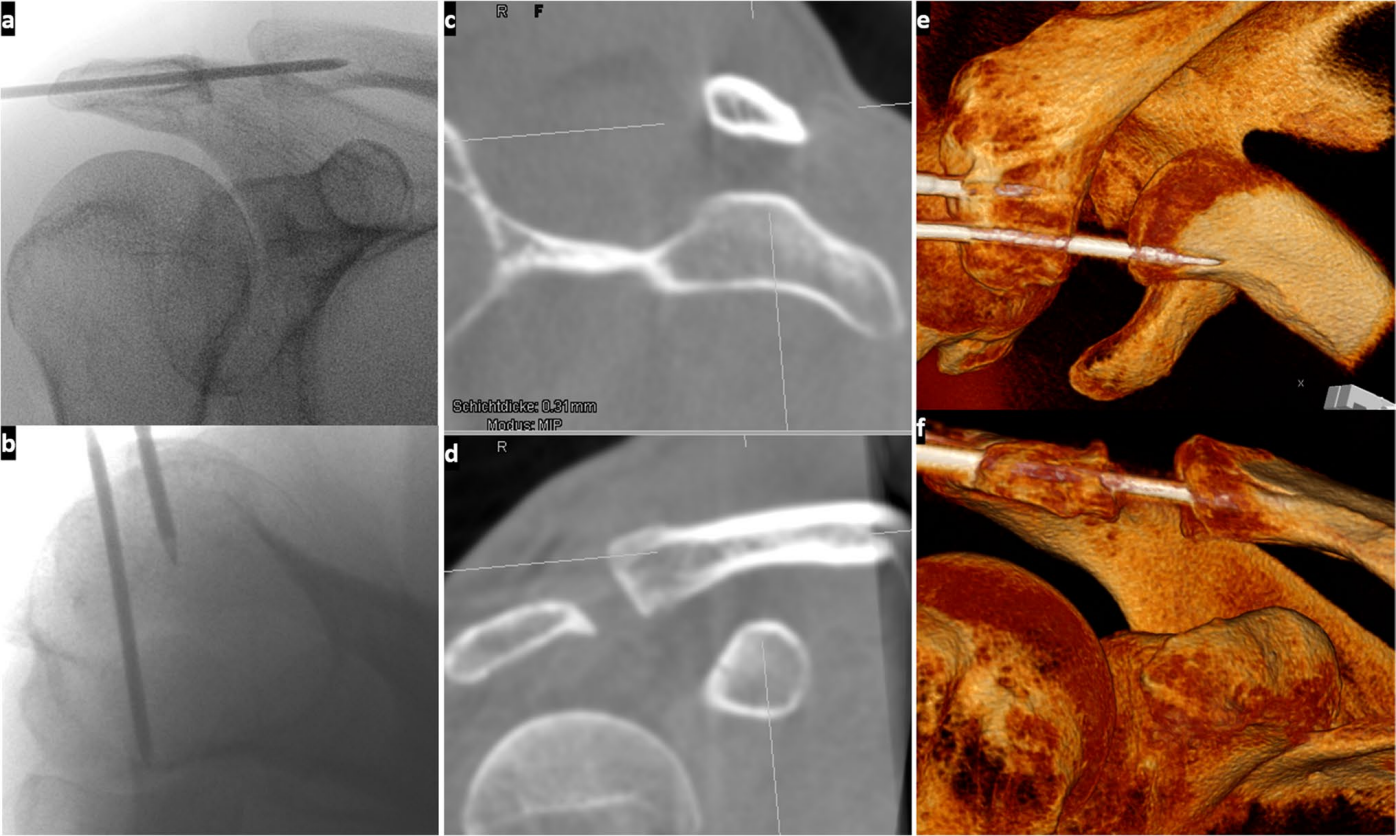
X-ray images after closed AC joint reduction and Kirschner wire retention are shown in a (coronal) and b (axial). The second wire is used to attach the first reference for computer navigation to the patient’s acromion. The second reference is located on the C-arm (see Fig. 1). In c and d parasagittal and coronal DVT slices can be seen, in e and f 3D reconstructions for exact assessment of the AC joint position
